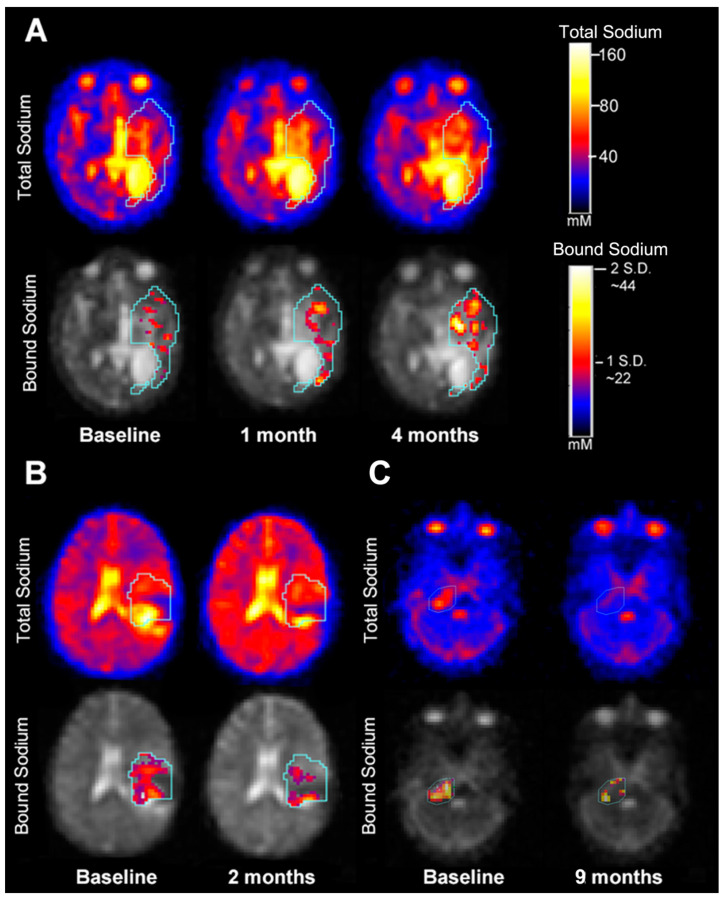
Two-TE sodium MRI scans showing tumor progression in high-grade glioma (Panel (A) [pt. ID 4027]), and response to therapy in supratentorial astrocytoma (Panel (B) [pt. ID 4010]) and in high-grade glioma (Panel (C) [pt. ID 4025). In the tumor regions of the bound sodium images are pixels of vBSC with a value greater than 1 standard deviation (S.D.) from the average vBSC value (~22 mM) over the tumor.
